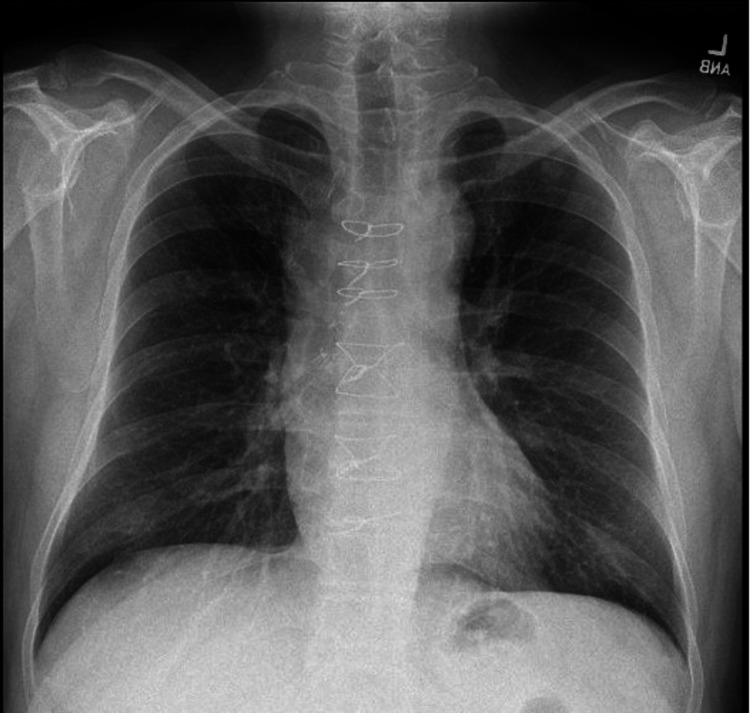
Repeat posteroanterior chest radiograph demonstrating wider upper mediastinum.
